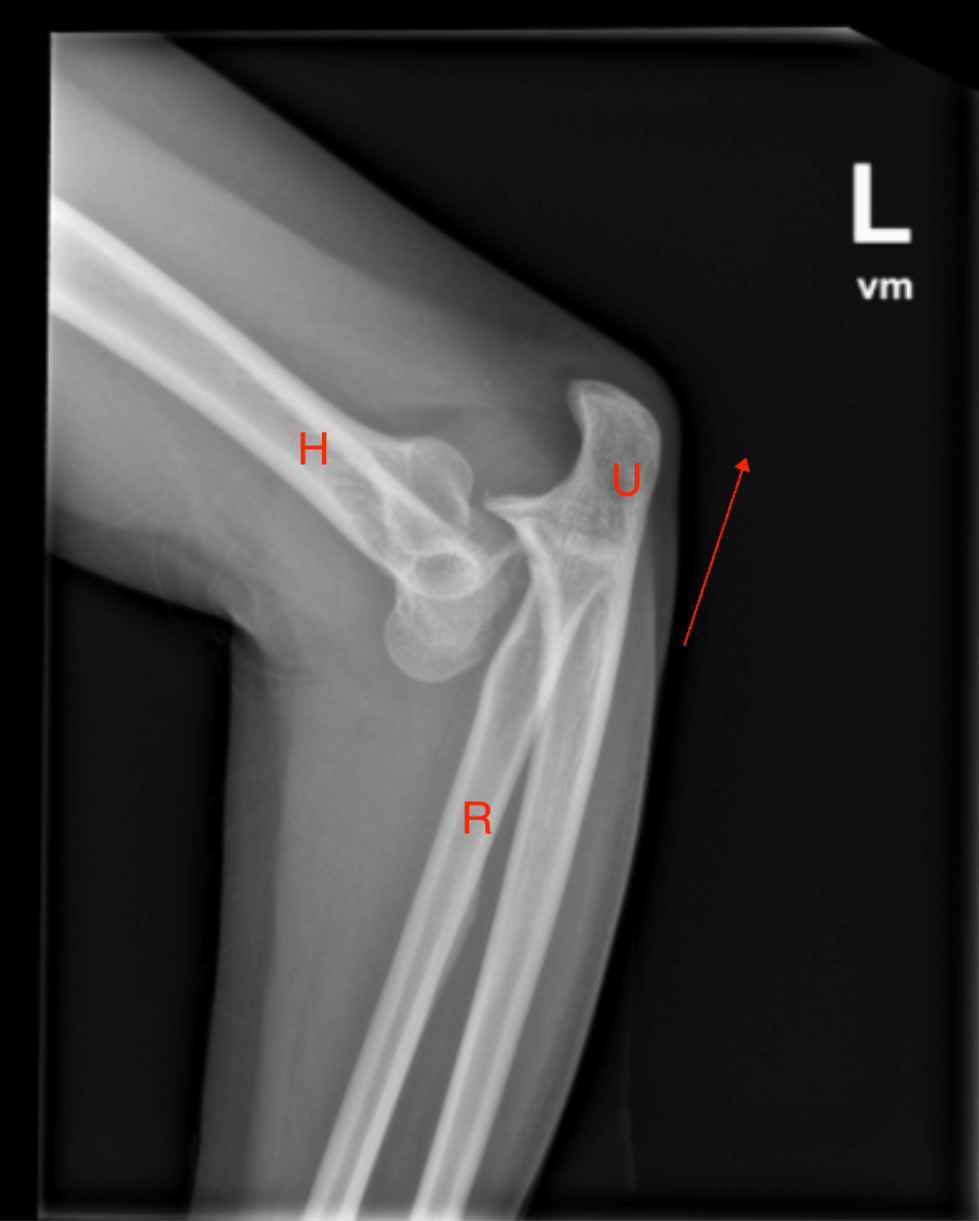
An X-ray of the left elbow before reduction showing posterolateral dislocation in the direction of the arrow. H: humerus; R: radius; U: ulna
